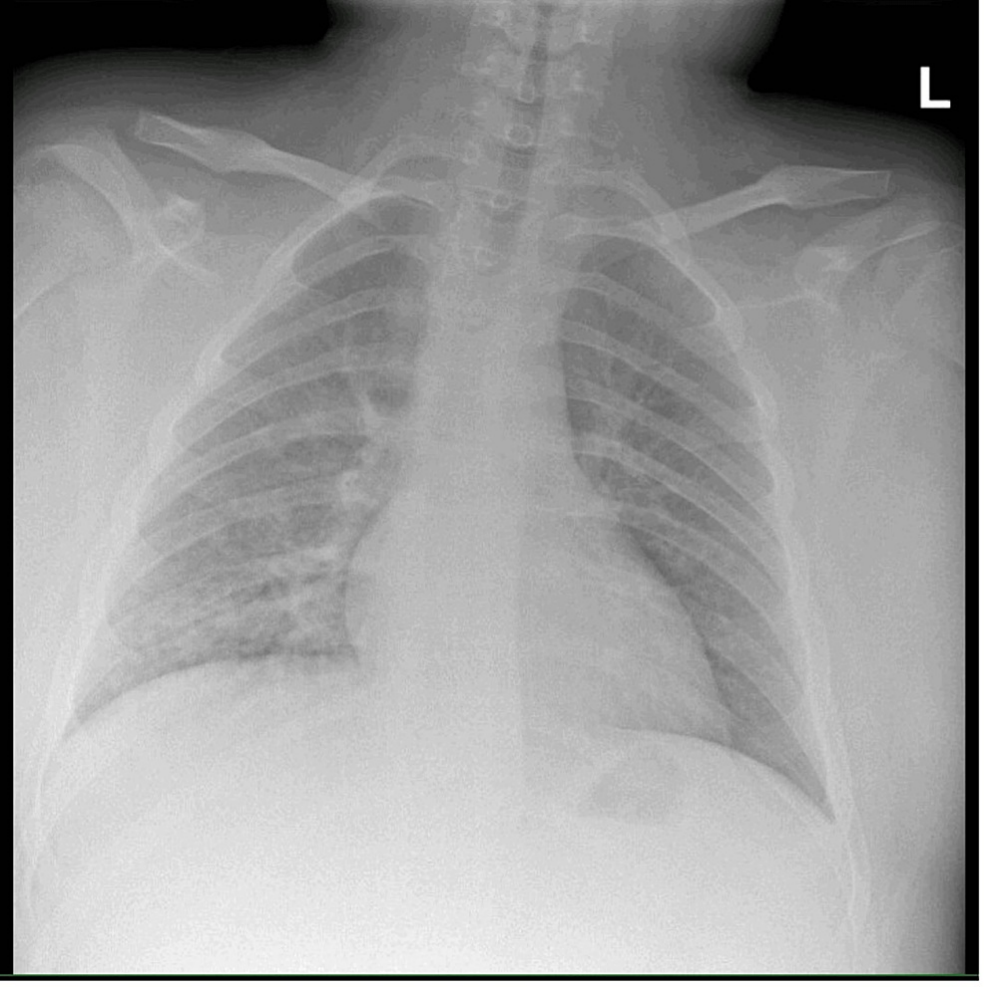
Anteroposterior chest radiograph of the patient.
